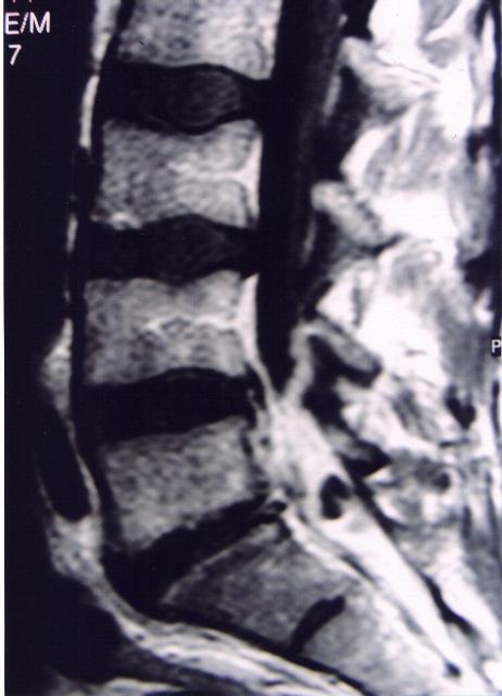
Following Gd administration, a sagittal T1-weighted image shows a marked enhancement of the L5 and S1 vertebral bodies, the L5-S1 inter-vertebral disk and the epidural fluid collection, suggesting spondylodiskitis with abscess formation at the L5-S1 level.
